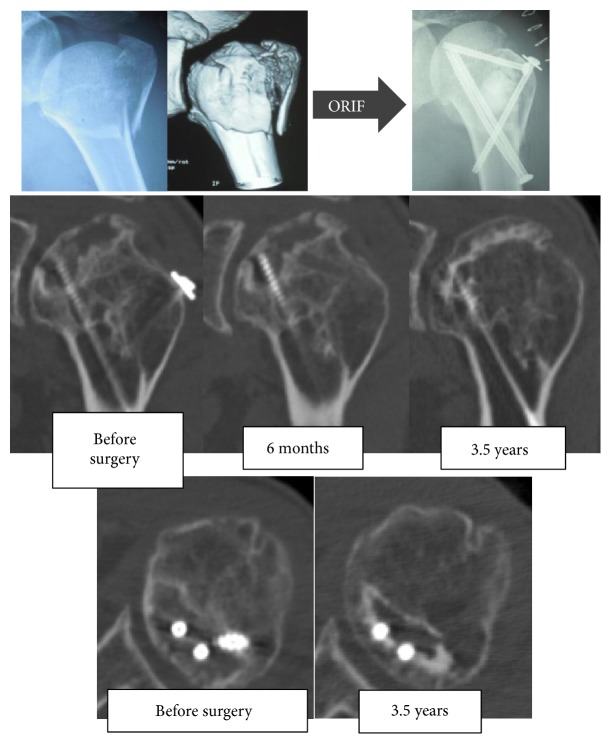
X-ray and computed tomography images of Case 2.
